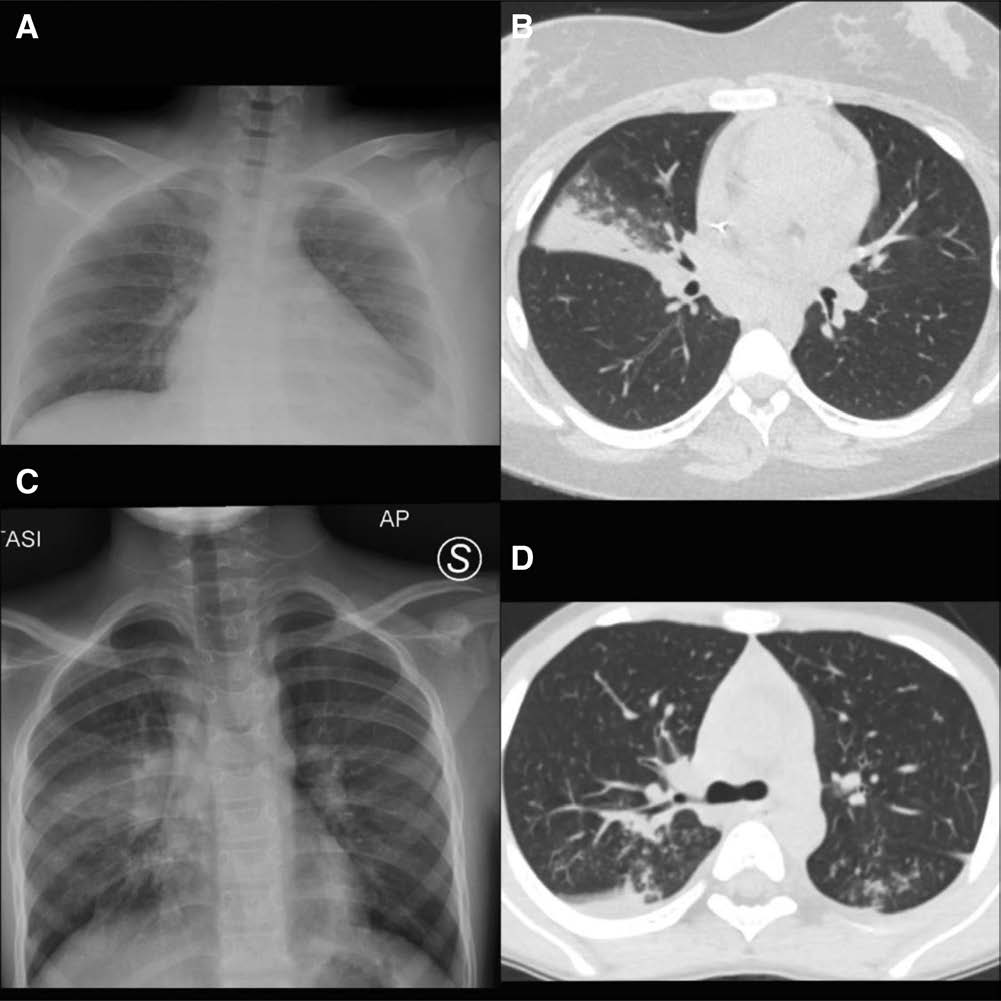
Radiologic findings in 2 patients. A: Chest radiograph showing left pleural effusion associated with basal consensual parenchymal hypoventilation; (B) chest CT scan revealing a ribbon-like area of parenchymal consolidation with air bronchogram. This formation is surrounded by several areas of slightly increased density of the pulmonary parenchyma, with ground-glass appearance, with multiple centrilobular micronodules some of which had a tree-in-bud aspect. In the right superior lobe, some calcified nodular formations are described, associated with calcified lymph nodes, compatible with the primary complex. Furthermore, there are several mediastinal lymphadenopathies. C: Chest radiograph showing a right hilar round-shaped parenchymal hypoventilation, surrounded by another medio-basal hypoventilation; (D) Chest CT scan revealing several bilateral areas of parenchymal consolidation, with irregular margins and a confluent appearance, and partially obstructed bronchi; several mediastinal lymphadenopathies, some of which characterized by internal necrosis. CT indicates computed tomography.
